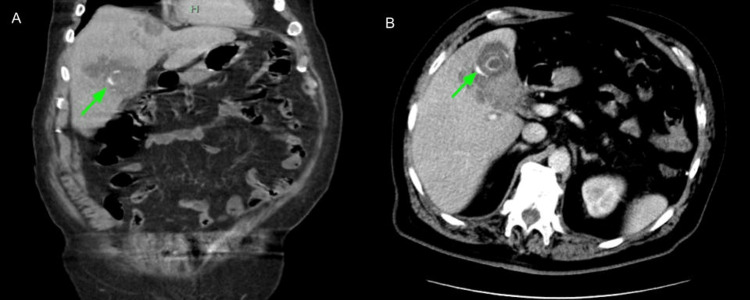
CT abdomen and pelvis with contrast shows multiple large gallstones within the gallbladder (green arrow in A - coronal view, B - axial view).
